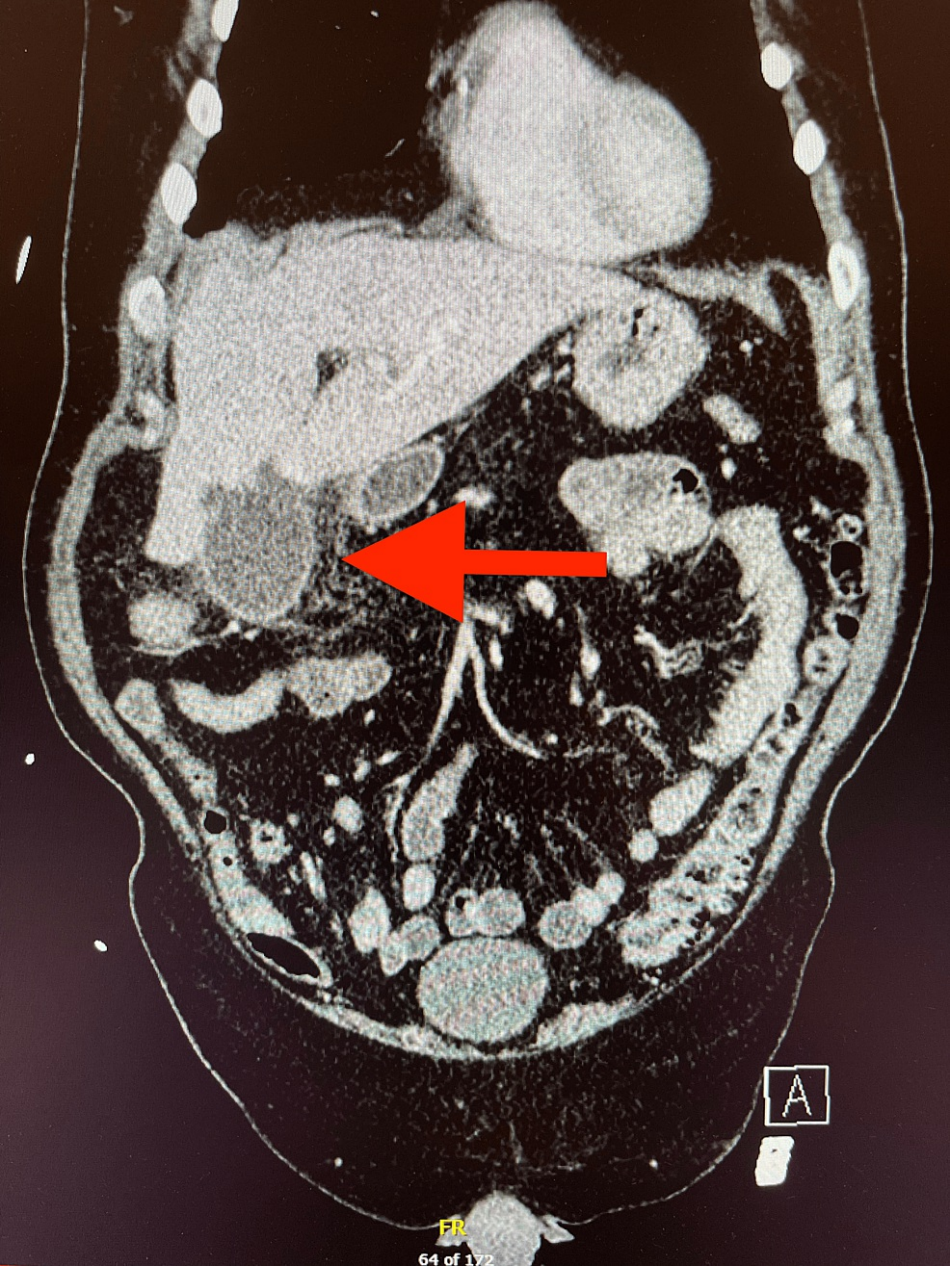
CT scan of the abdomen with and without contrast The arrow points to show perforated gallbladder.
